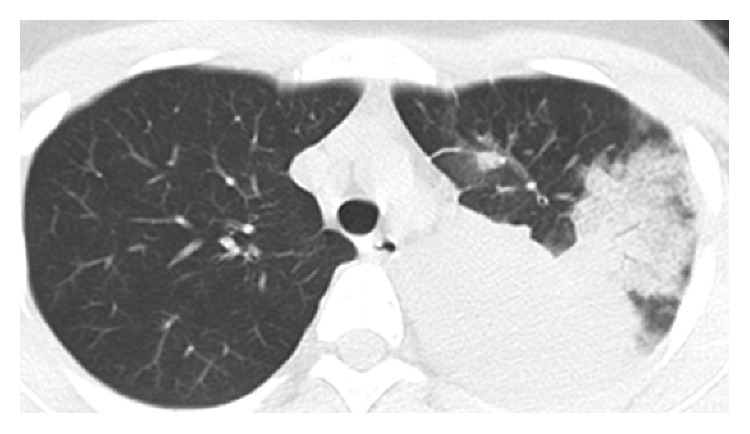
Chest computed tomography image on admission showing consolidation with an air bronchogram in the left upper lobe.
